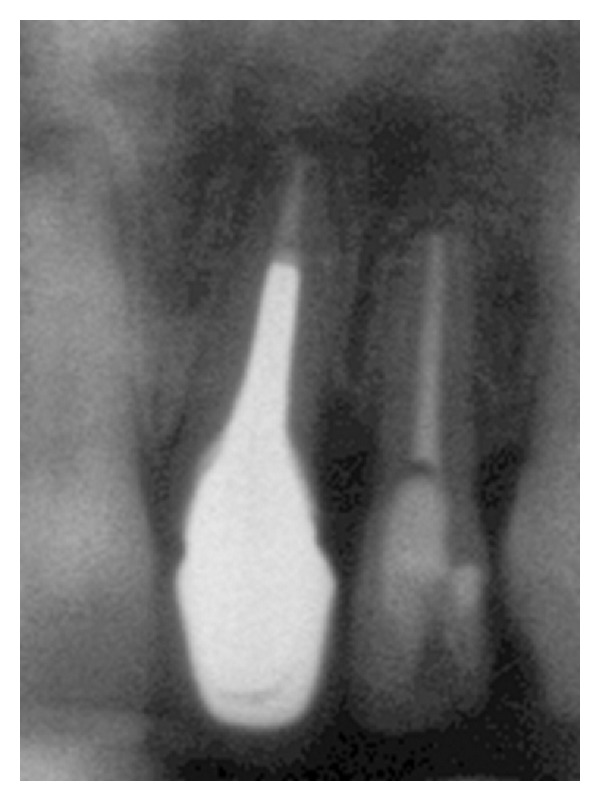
Initial radiograph of maxillary left central incisor.
